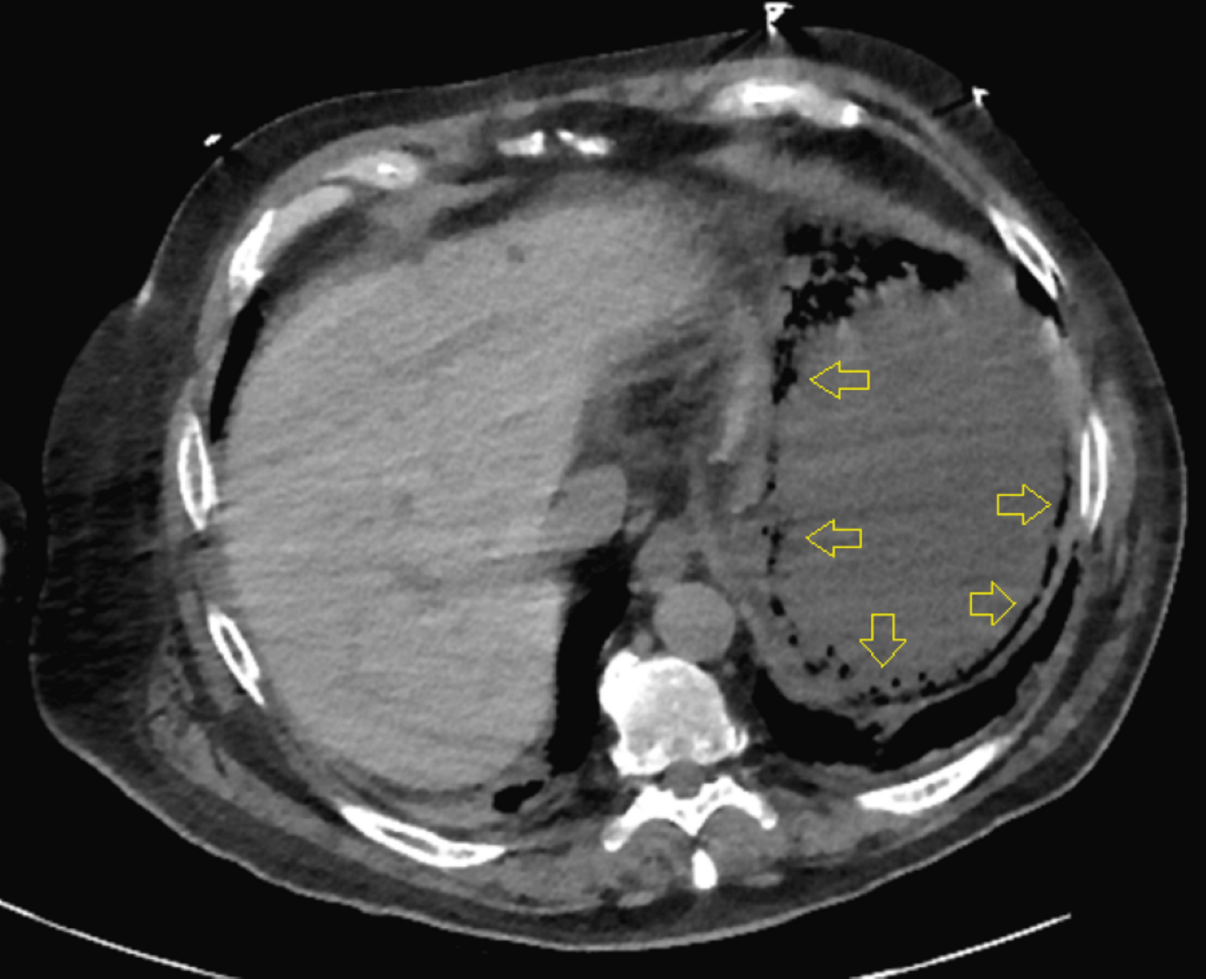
Initial computed tomography imaging demonstrating emphysematous gastritis The arrows indicate the emphysematous gastritis.
